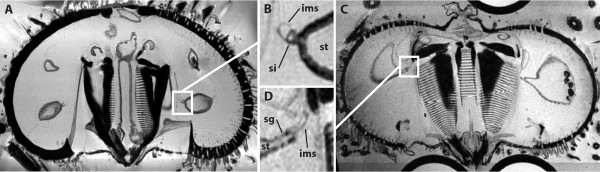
Magnetic resonance imaging: potential and limitations for comparative morphological analyses. Vertical MRI sections of two sea urchin (Echinoidea) species passing from pharynx to periproct. A *Psammechinus miliaris *(Müller, 1771), (44 μm)^3 ^dataset resolution, contrast agent: Magnevist. B Inset from A showing the stomach (st) with adjacent siphon (si) and inner marginal sinus (ims). C *Diadema savignyi *Michelin, 1845, (40 μm)^3 ^dataset resolution, contrast agent: Magnevist. D Inset from C showing the stomach (st) with siphonal groove (sg) and adjacent inner marginal sinus (ims). Although both scans permit to differentiate various minute and large structures, the inner marginal sinus (ims) present in *D. savignyi *can be mistaken for a siphon. Vertical sections and magnifications were generated using the Volume Viewer in ImageJ 1.41o.
